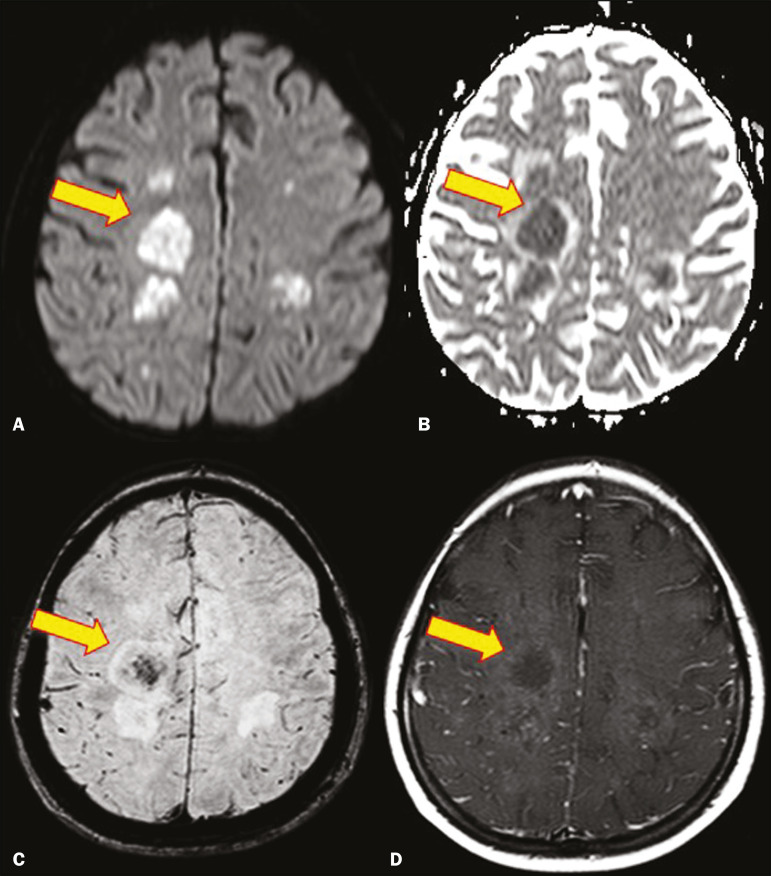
A 13-year-old immunocompromised patient with invasive aspergillosis of the CNS. A,B: Axial DWI and apparent diffusion coefficient map (arrows), respectively, showing restricted diffusion; C: Susceptibility-weighted imaging showing hemosiderin deposition (arrow); D: Contrastenhanced axial T1-weighted MRI sequence showing slight peripheral enhancement (arrow). Findings typical of fungal lesions caused by *Aspergillus* sp.
